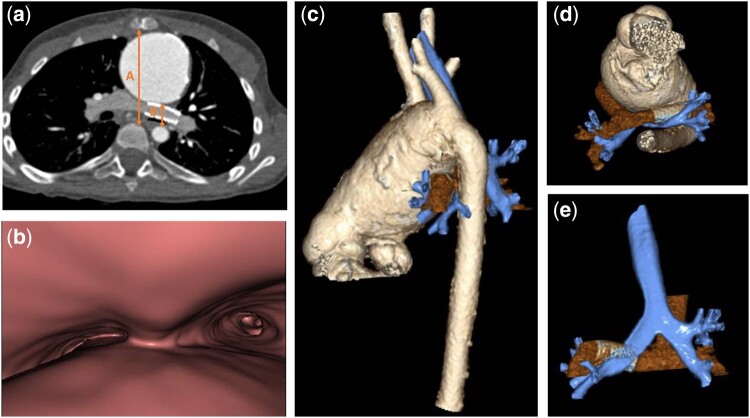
Preoperative computed tomography. Axial contrast-enhanced computed tomography image (a) with virtual bronchoscopy (b) and 3-dimensional volume rendering reconstructions (c–e) show a severely enlarged ascending aorta with posterior displacement of the stented left pulmonary artery and marked left main bronchus compression. The interaortic space from the posterior margin of the ascending aorta to the anterior margin of the descending aorta (distance *B* in a) is critically narrowed, with a low value of the interaortic ratio (*B*/*A*).
